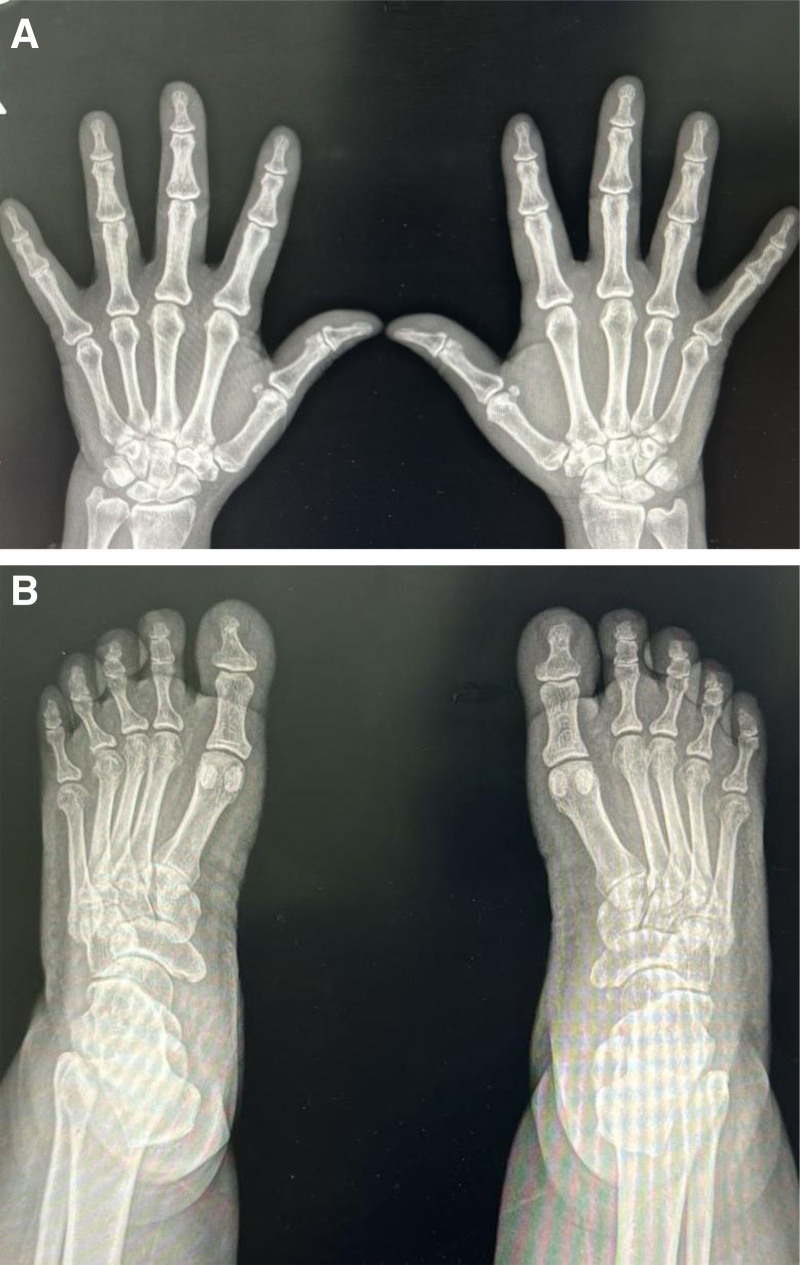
Hand and foot radiographs.
